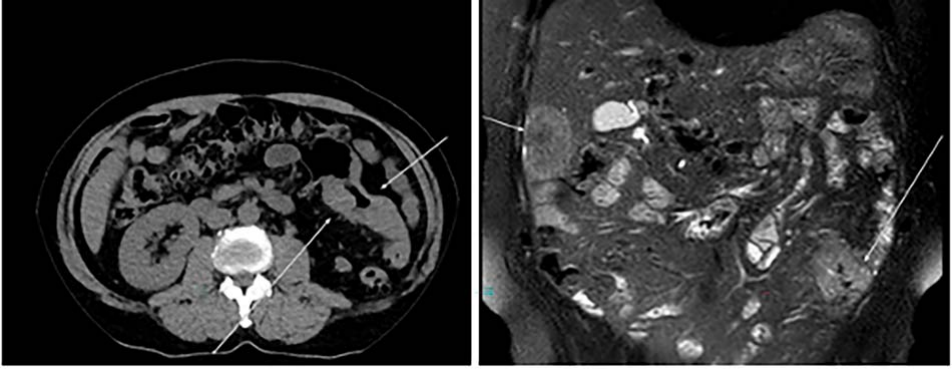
CT: status of pre administration of NAC.
